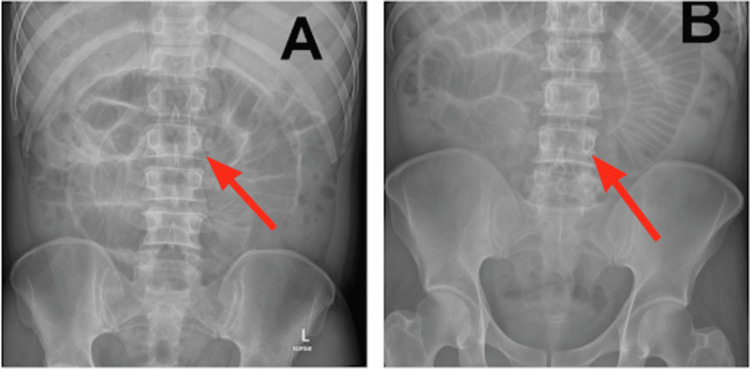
Upright abdominal X-ray A, B: dilated bowel loops with plicae circulares, consistent with small bowel obstruction. Red arrows highlight plicae circulares, consistent with dilated small bowel.
